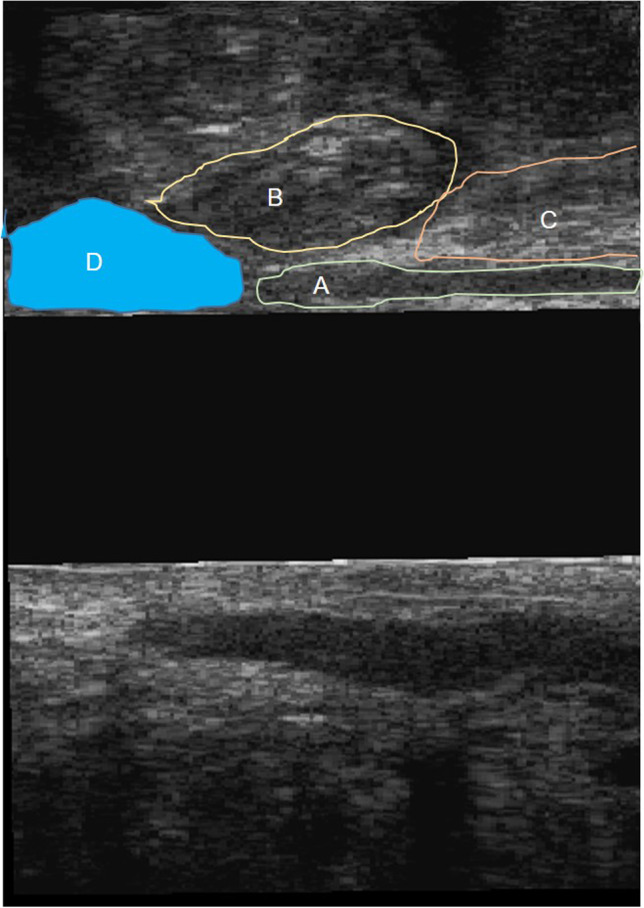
Endoanal ultrasound image showing a sagittal section of an anterior post-fistulotomy external and internal anal sphincter defects and remaining sphincters. A internal anal sphincter, B external anal sphincter, C puborectalis muscle, D fistulotomy defect
